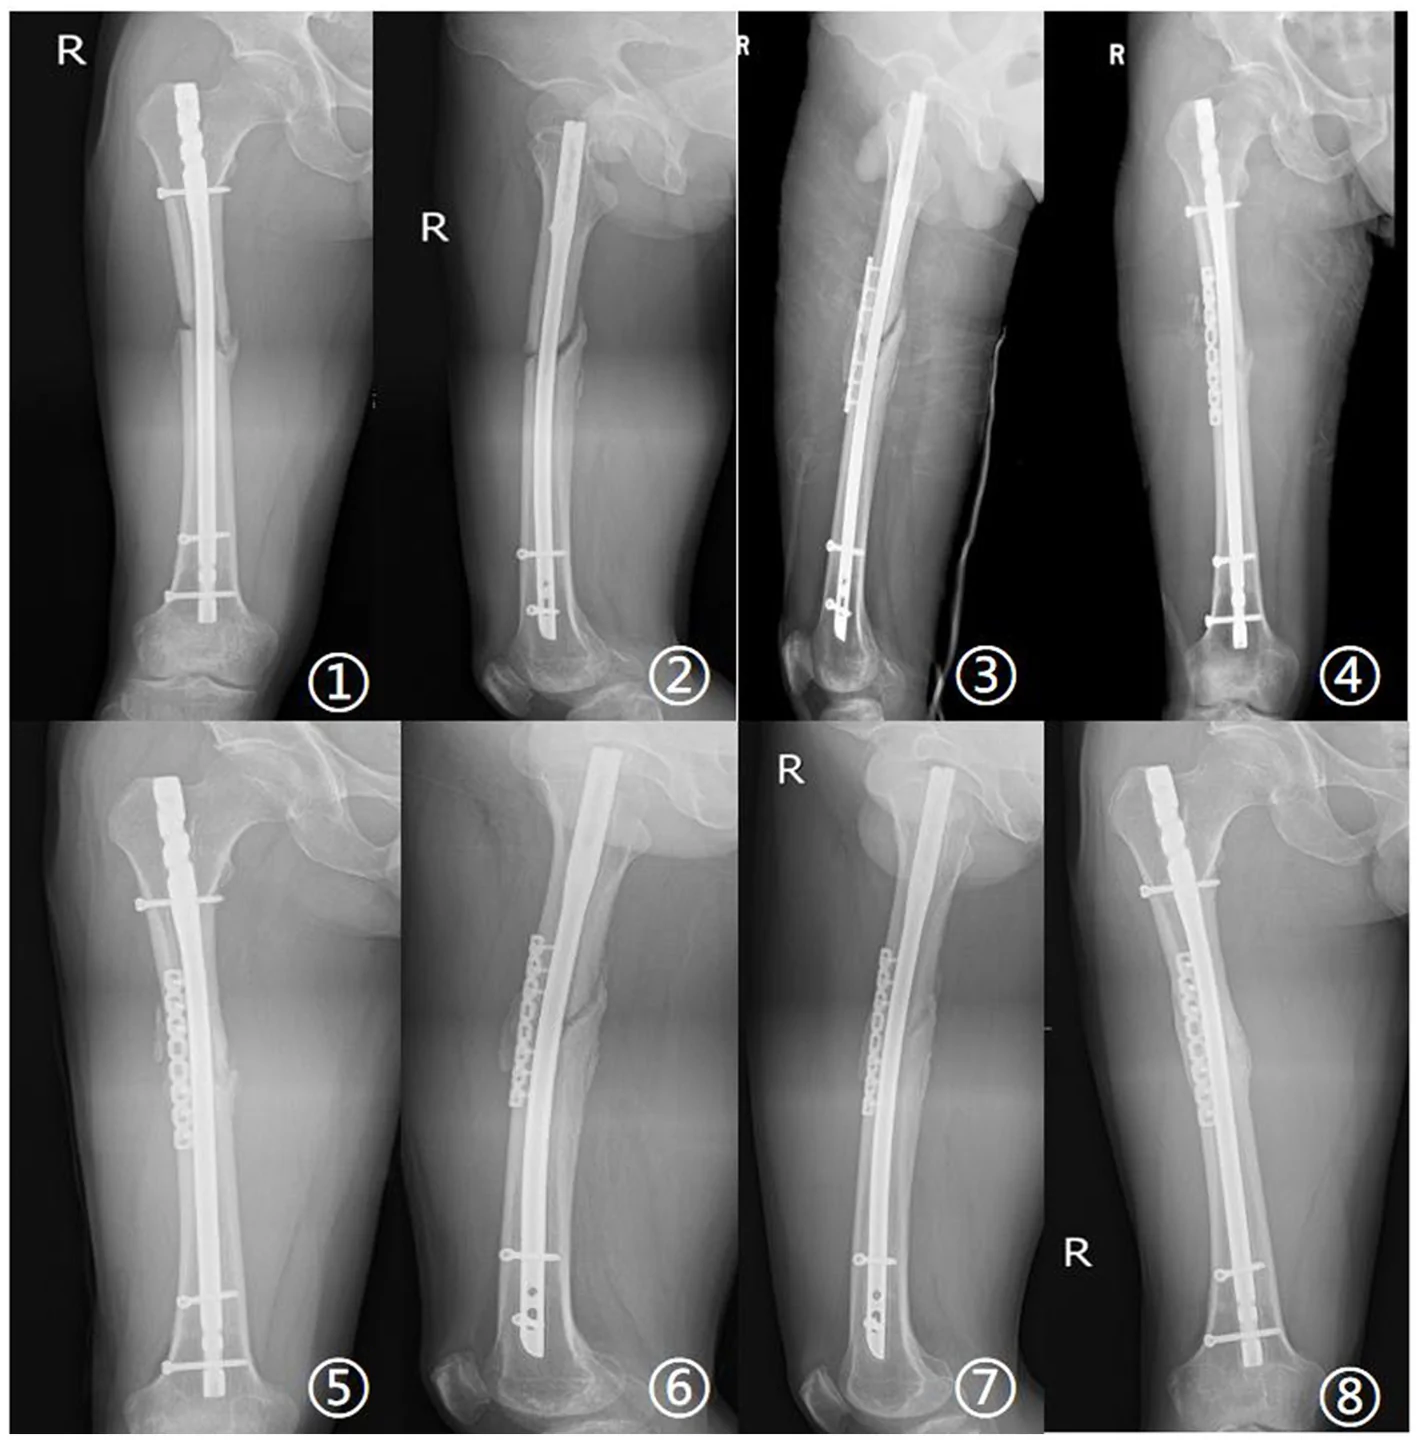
Standard open augmentative plating for hypertrophic femoral non-union. (1, 2) Pre-operative anteroposterior (AP) and lateral radiographs showing a typical hypertrophic non-union after reamed femoral IMN. An abundant “elephant foot” callus is visible at the fracture ends, indicating an active biological response that failed to bridge due to mechanical instability. (3, 4) Immediate post-operative AP and lateral radiographs. An extensive lateral approach was used to apply a lateral locking compression plate. This “maximal fixation” strategy provided absolute stability at the expense of extensive soft tissue stripping. (5, 6) Follow-up radiographs at 3 months showing gradual callus remodeling and bridging across the fracture line. (7, 8) Radiographs at 6 months showing the complete disappearance of the fracture line, achieving solid bone union.
